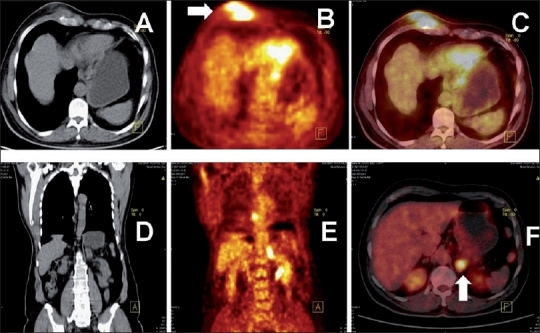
Tuberculosis of chest wall and adrenal gland. Axial (A) and coronal (D) plain CT, PET (B, E), and axial PET/CT (C, F) images in a 57-year-old man with an anterior right chest wall swelling shows intense subcutaneous FDG uptake within a soft tissue mass involving the fi fth costochondral junction, associated with increased FDG uptake in an enlarged left adrenal gland (arrows). A soft tissue chest wall sarcoma with adrenal metastasis was suspected. Biopsy of the chest wall lesion revealed TB. The lesions regressed following anti-tuberculous therapy
